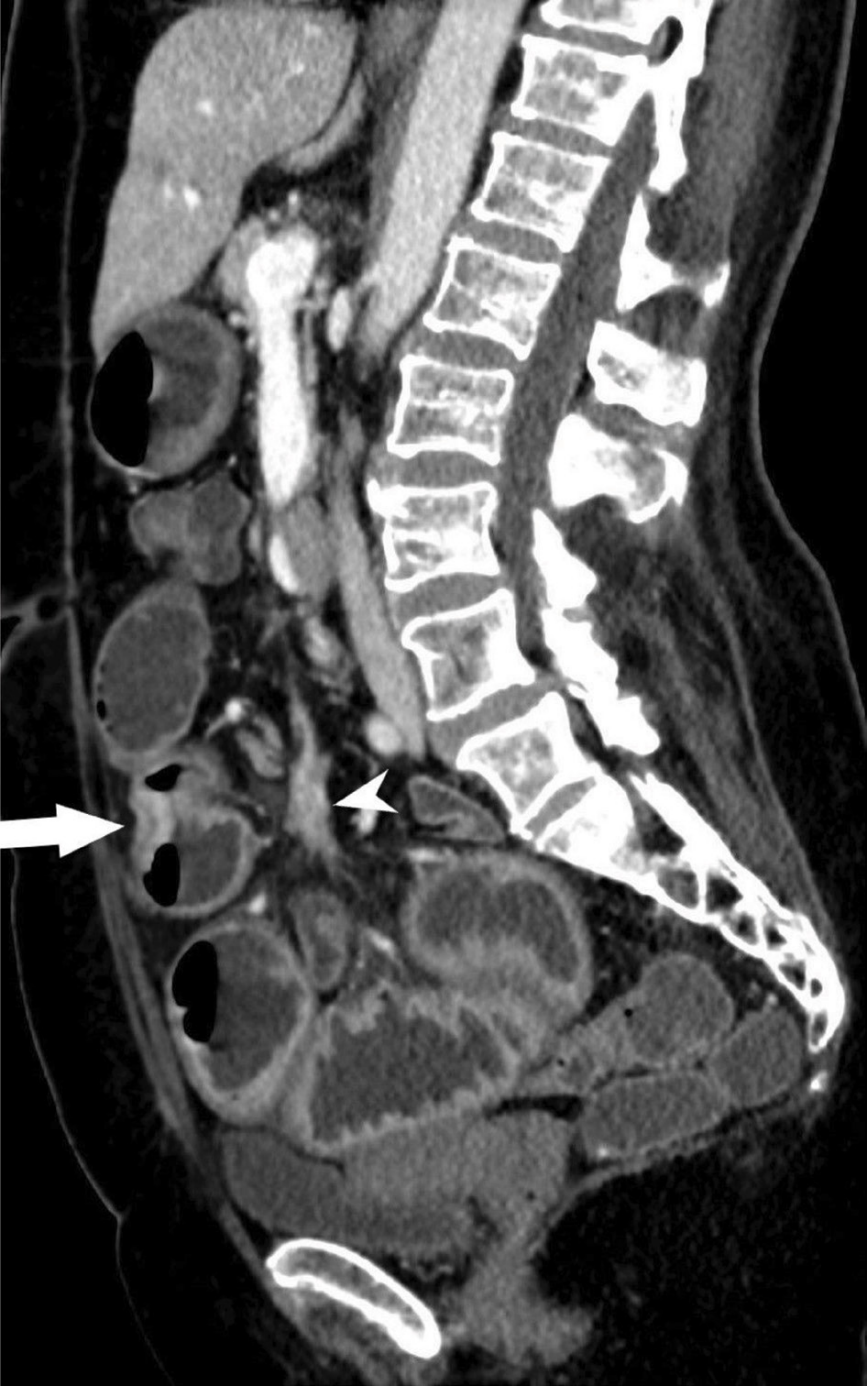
CT demonstrating stenotic ileal NET with adjacent mesenteric mass.
